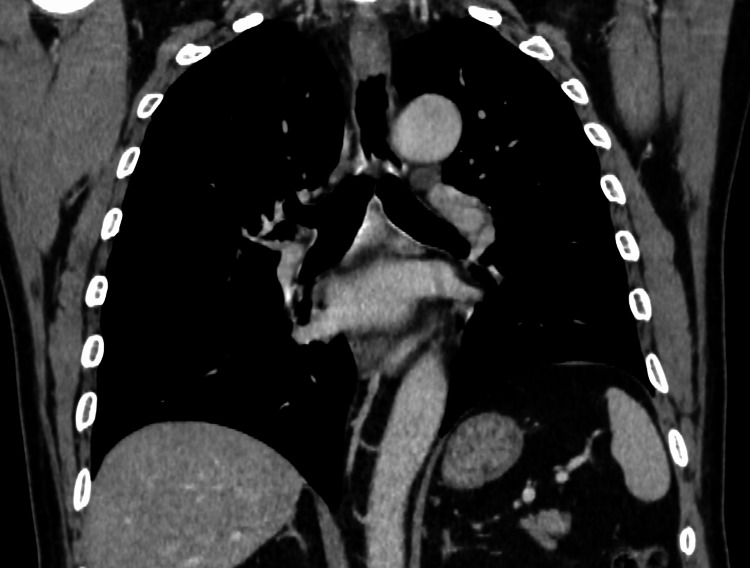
Coronal post-contrast CT showing no acquired obstructive lesion Coronal contrast-enhanced chest CT reconstruction demonstrating the absence of an enhancing endobronchial lesion, suspicious pulmonary mass, post-obstructive consolidation, and significant mediastinal or hilar lymphadenopathy.
